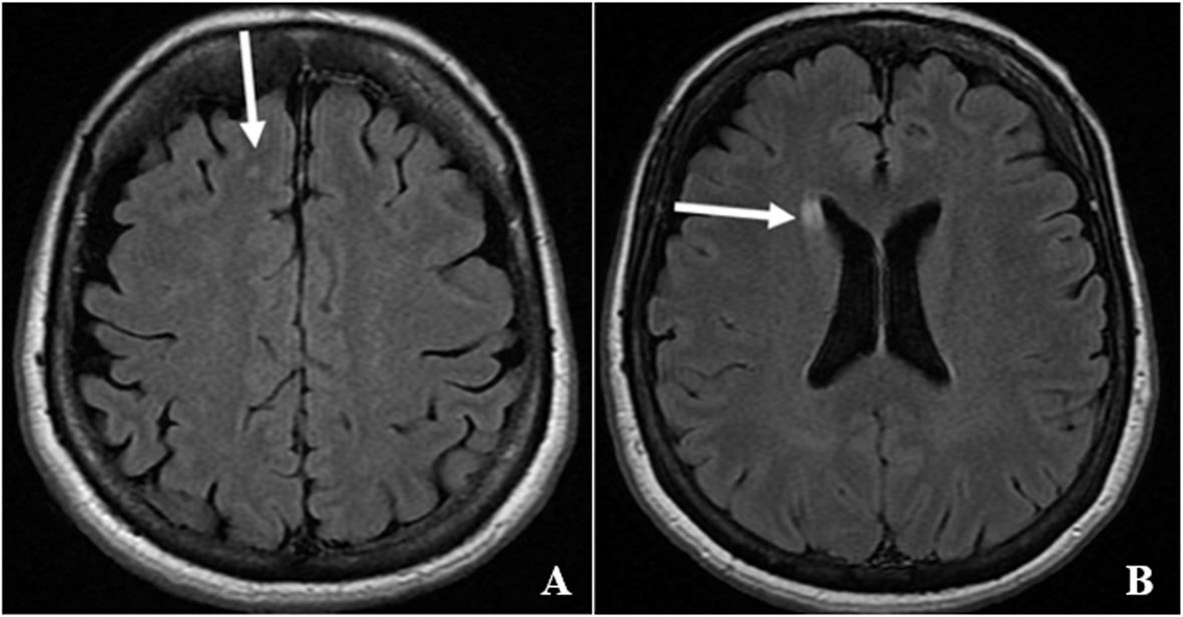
Transverse T2-FLAIR magnetic resonance images of the brain showing probable embolic lesions (white arrows) of variable age in the A right frontal lobe, and B right caudate head
